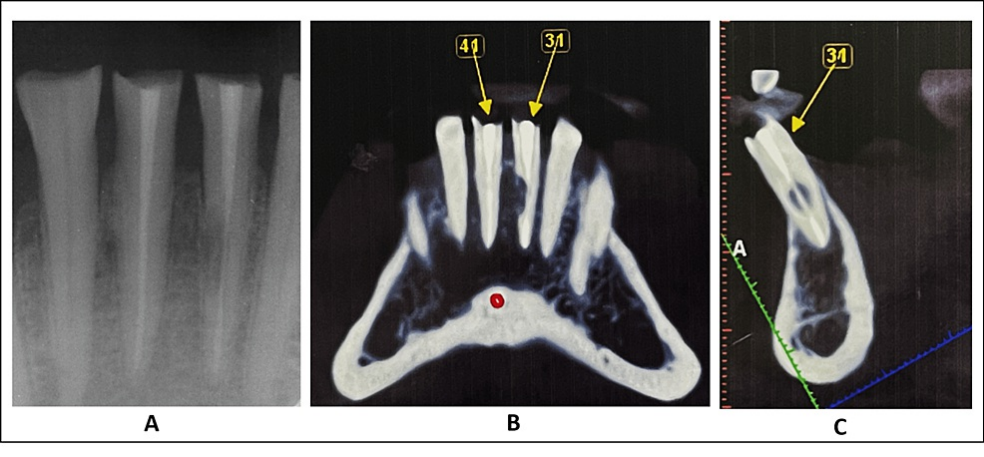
Preoperative assessment. A: Preoperative intraoral periapical radiograph. B: Cone-beam computed tomography slice of teeth #31 and #41. C: Sagittal view of internal root resorption in tooth #31.
